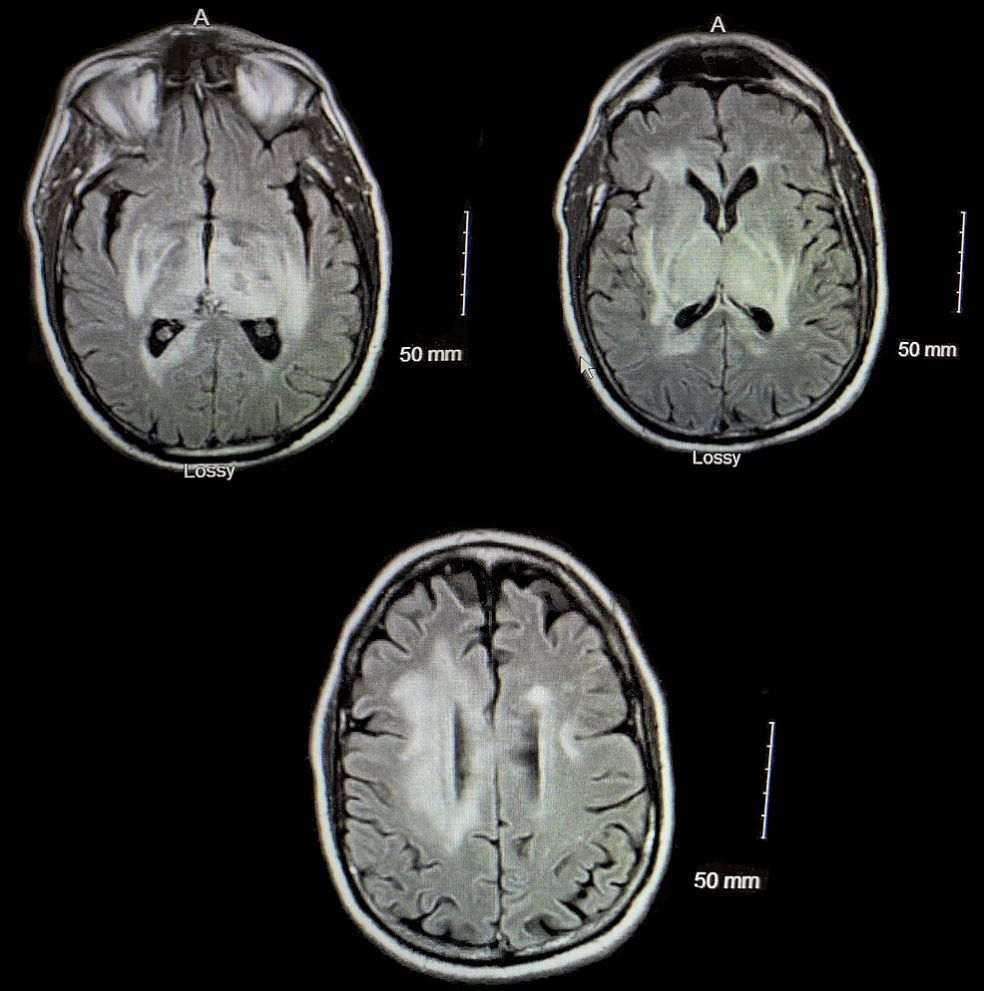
MRI of the brain showing diffuse edema and micro-hemorrhages.
